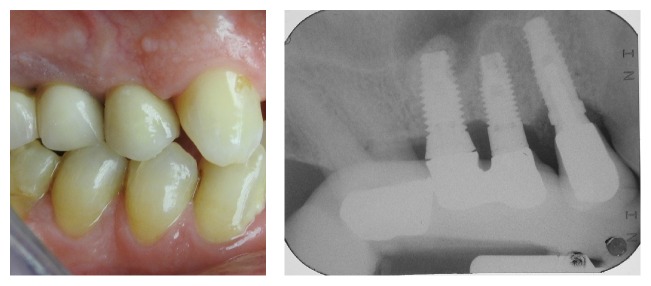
Case 1: definitive prosthetic restoration with periapical radiograph.
